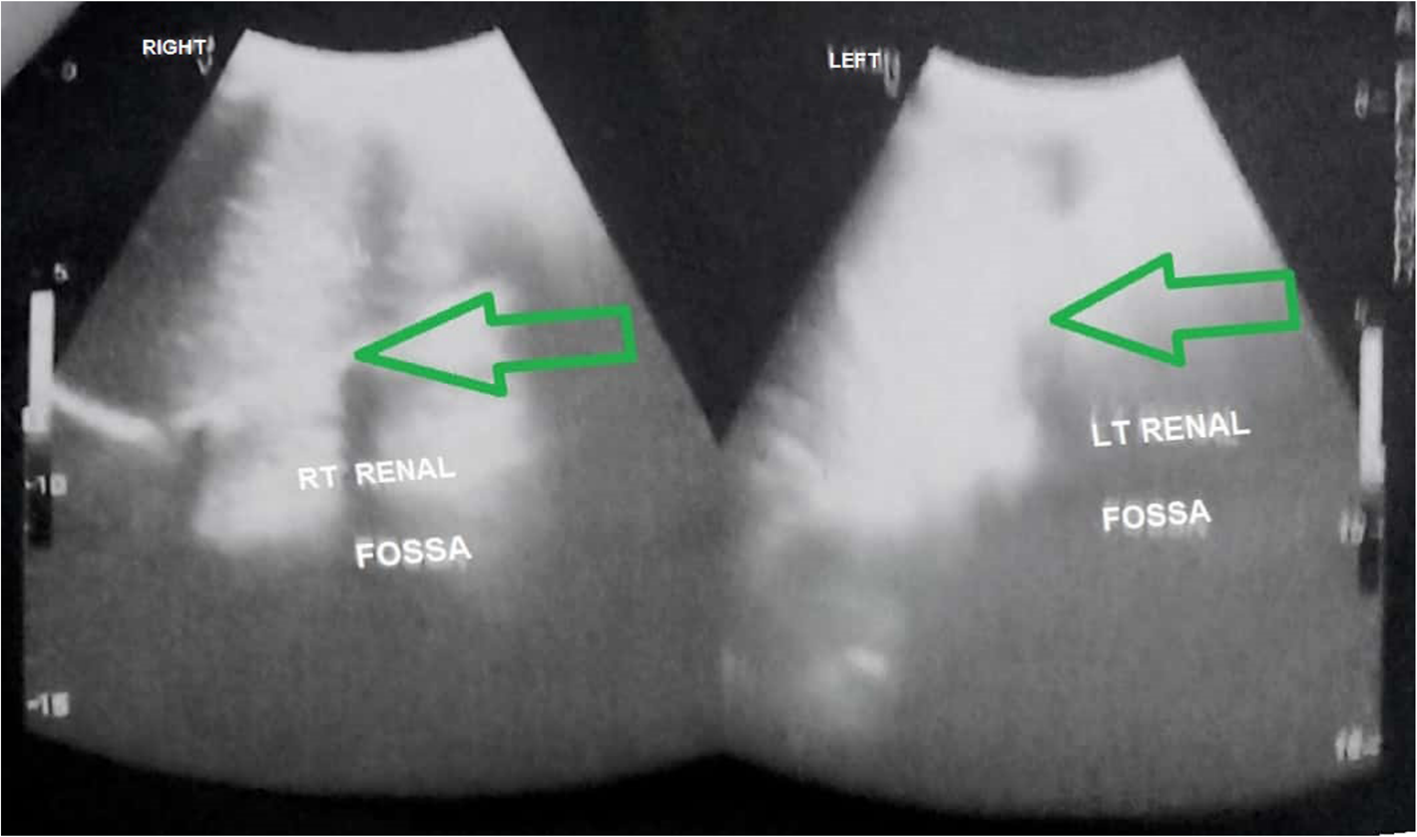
Grey scale ultrasonography scan of the abdomen shows bilateral empty renal fossa (*green arrows*)
